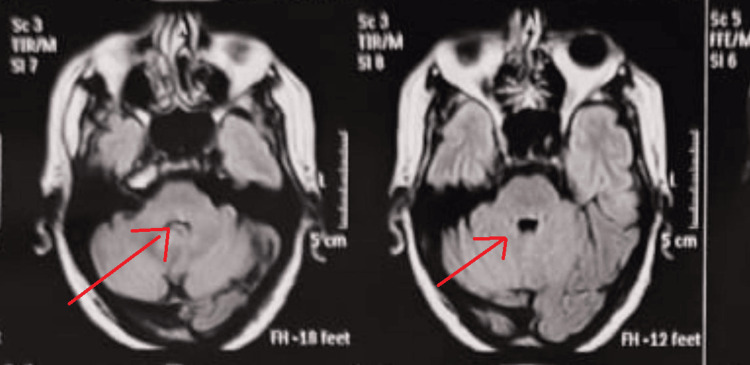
MRI demonstrates a small diffusion-restricted infarct in the left dorsal pons, hyperintense on DWI/FLAIR with corresponding ADC hypointensity and no hemorrhage. While individual brainstem nuclei are not distinctly visualized, the lesion location supports the clinical diagnosis of eight-and-a-half syndrome. DWI: diffusion-weighted imaging; FLAIR: fluid-attenuated inversion recovery; ADC: antibody–drug conjugate
